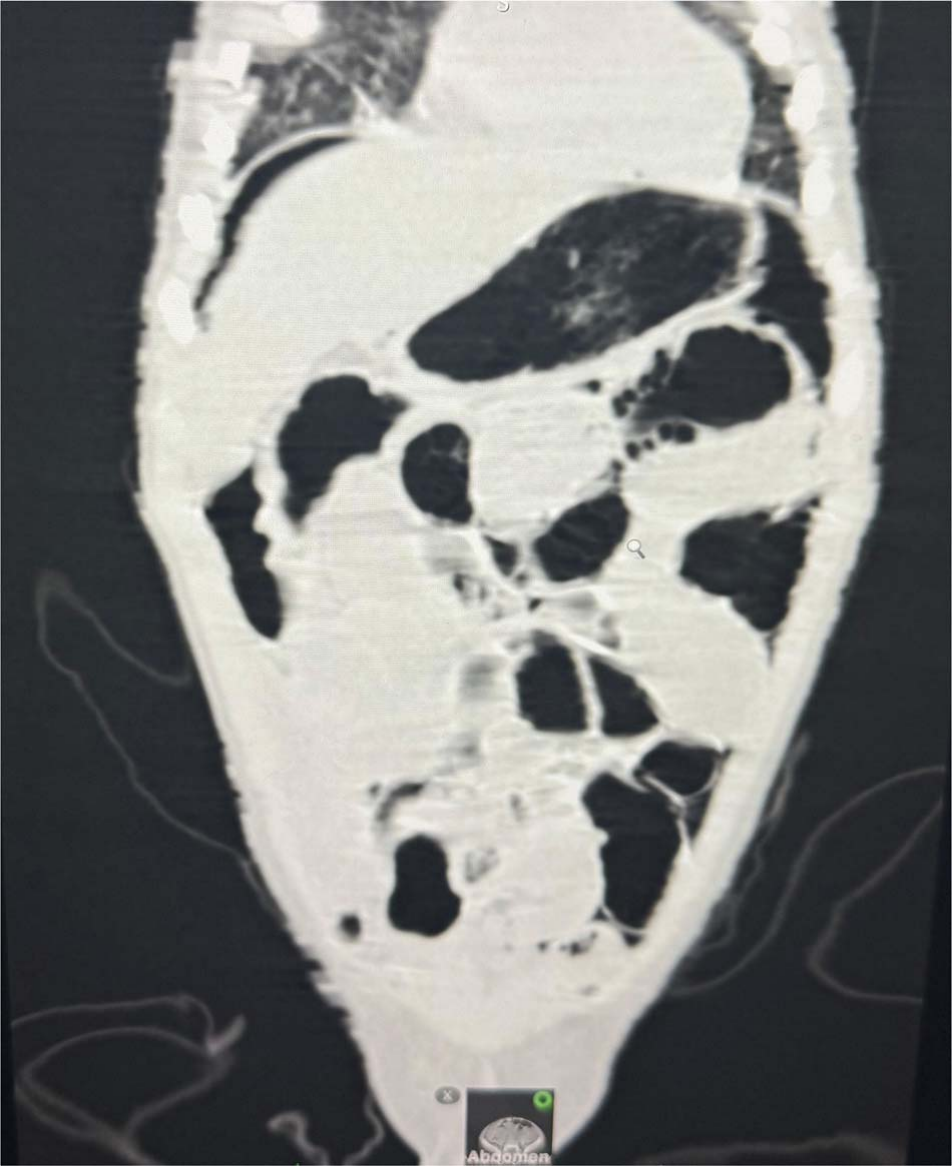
CT abdomen revealed free air under the diaphragm, indicating hollow viscus perforation.
